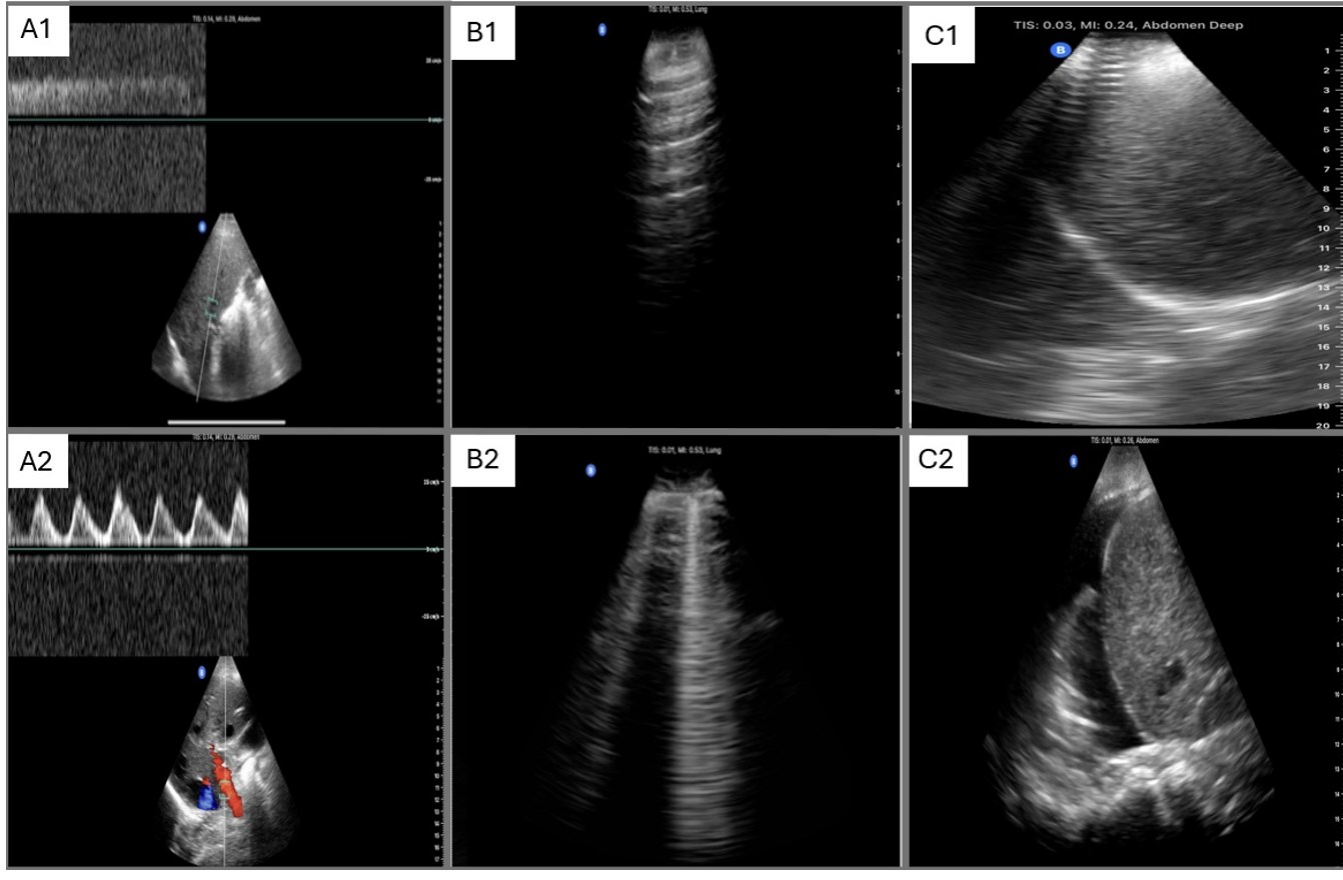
Point of care ultrasound (POCUS) evaluation of congestion.A. Portal vein pulsatility evaluated in the hepatic window with a pulsed wave Doppler. A1. Portal vein pulsatility less than 30%. A2. Portal vein pulsatility above 30%. B. Lung ultrasound. B1. A-lines in a lung without congestion. B2. B-lines in the context of Alveolar interstitial syndrome. C. Lung ultrasound for the evaluation of pleural effusion. C1. No pleural effusion. C2. Pleural effusion in the right lung.
